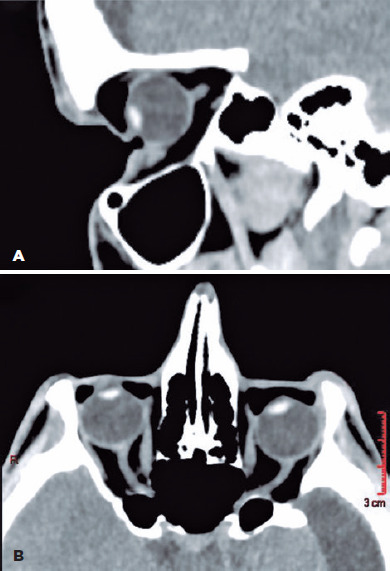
(A) Sagittal and (B) axial CT scans show localization of various abnormal
